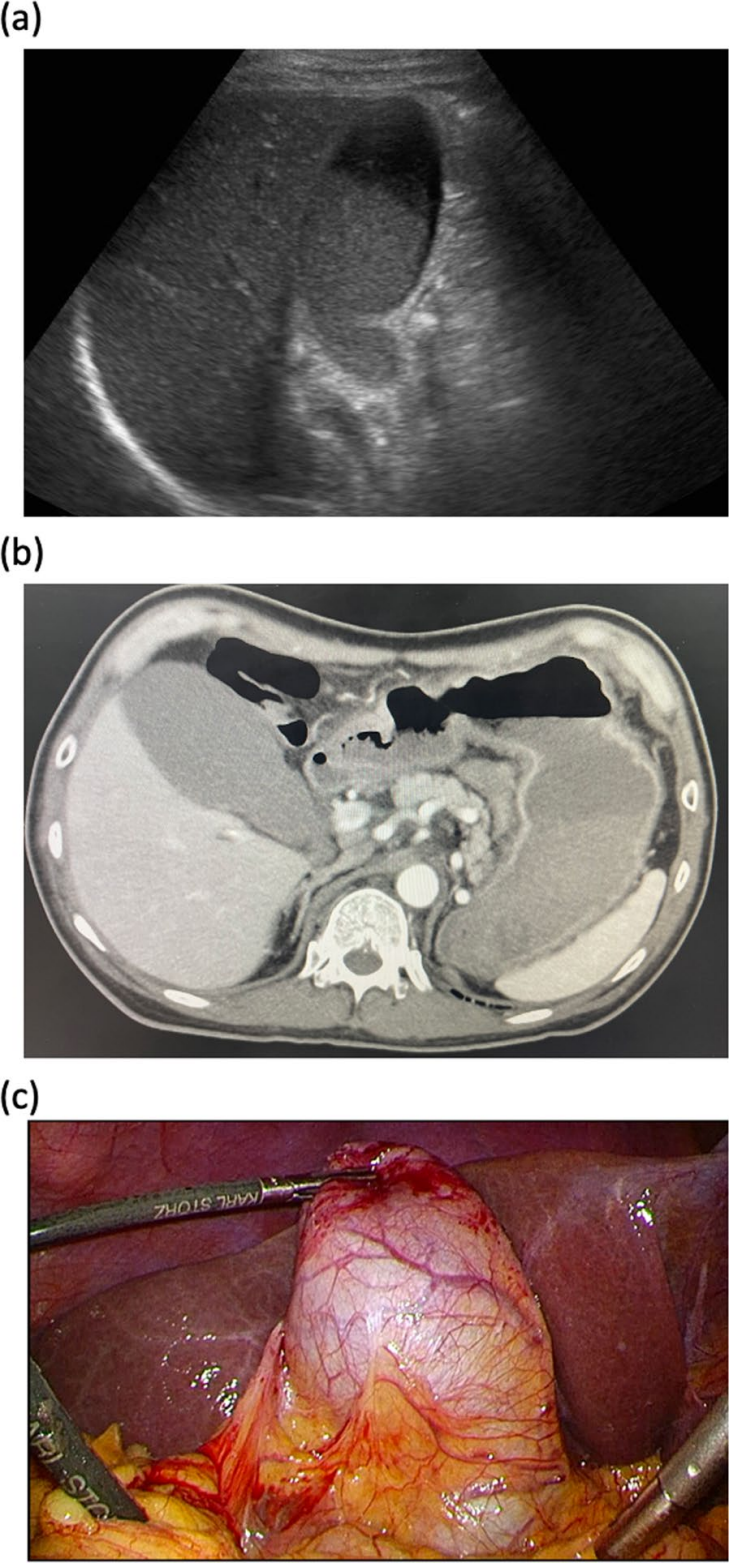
a Abdominal ultrasonography. No gallstones or wall thickening are observed. Bile sludge is detected in the gallbladder. Contrast-enhanced abdominal tomography. The gallbladder is mildly enlarged; however, no wall thickening or gallstone formation is observed. b Surgical findings. The gallbladder is mildly enlarged; however, no thickening is observed, and there is little evidence of neovascularization and tissue fragility
